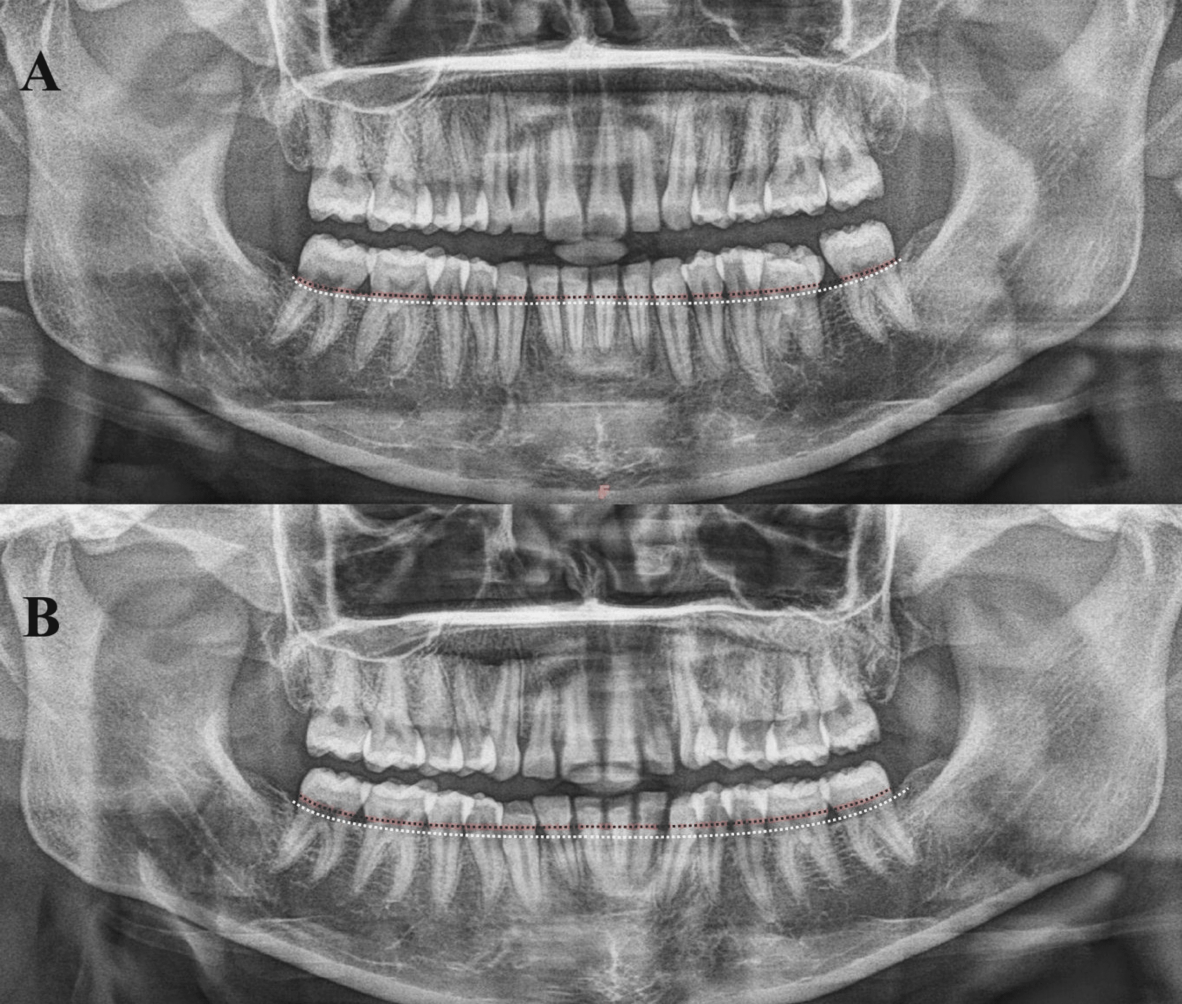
Panoramic radiograph of a patient pre- (A) and post-treatment with clear aligners These original OPG images of a patient from the study, used with permission, show the level of the alveolar bone crest (white interrupted line) and the level of the CEJ (red interrupted line) CEJ: Cementoenamel junction, OPG: Orthopantomogram
